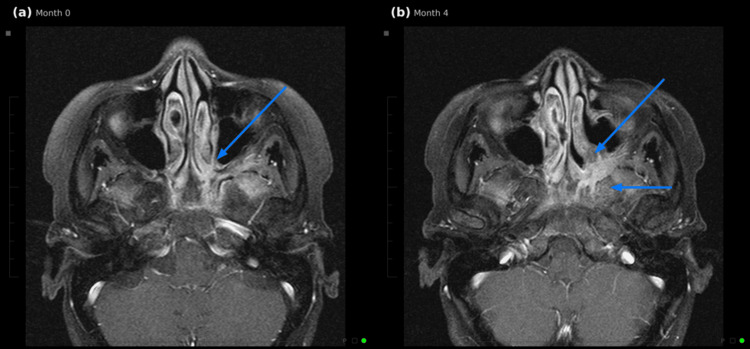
Axial post-gadolinium T1-weighted fat-saturated MRI demonstrating interval progression of perineural mucormycosis. (a) Month 0: Abnormal enhancing tissue within the left pterygopalatine fossa and retromaxillary region (arrow), consistent with infiltrative fungal disease. (b) Month 4: Interval progression with increased infiltration of the retromaxillary fat and medial and lateral pterygoid muscles (arrows), with persistent enhancement along the course of the vidian and maxillary nerves MRI: magnetic resonance imaging
